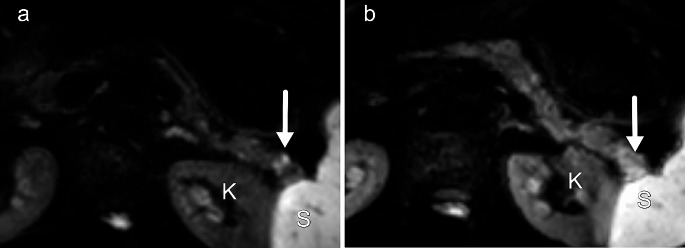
Diffusion-weighted image (b 800) of a small PDAC in the pancreatic tail. In July (a), a small focus of high signal was visible, but EUS was negative. Six months later (b) a larger area of high signal was visible. On pathology, the actual size of the tumor was only 6 mm, and the larger aspect of the tumor on DWI was due to inflammation and fibrosis around the tumor K = left kidney, S = spleen
